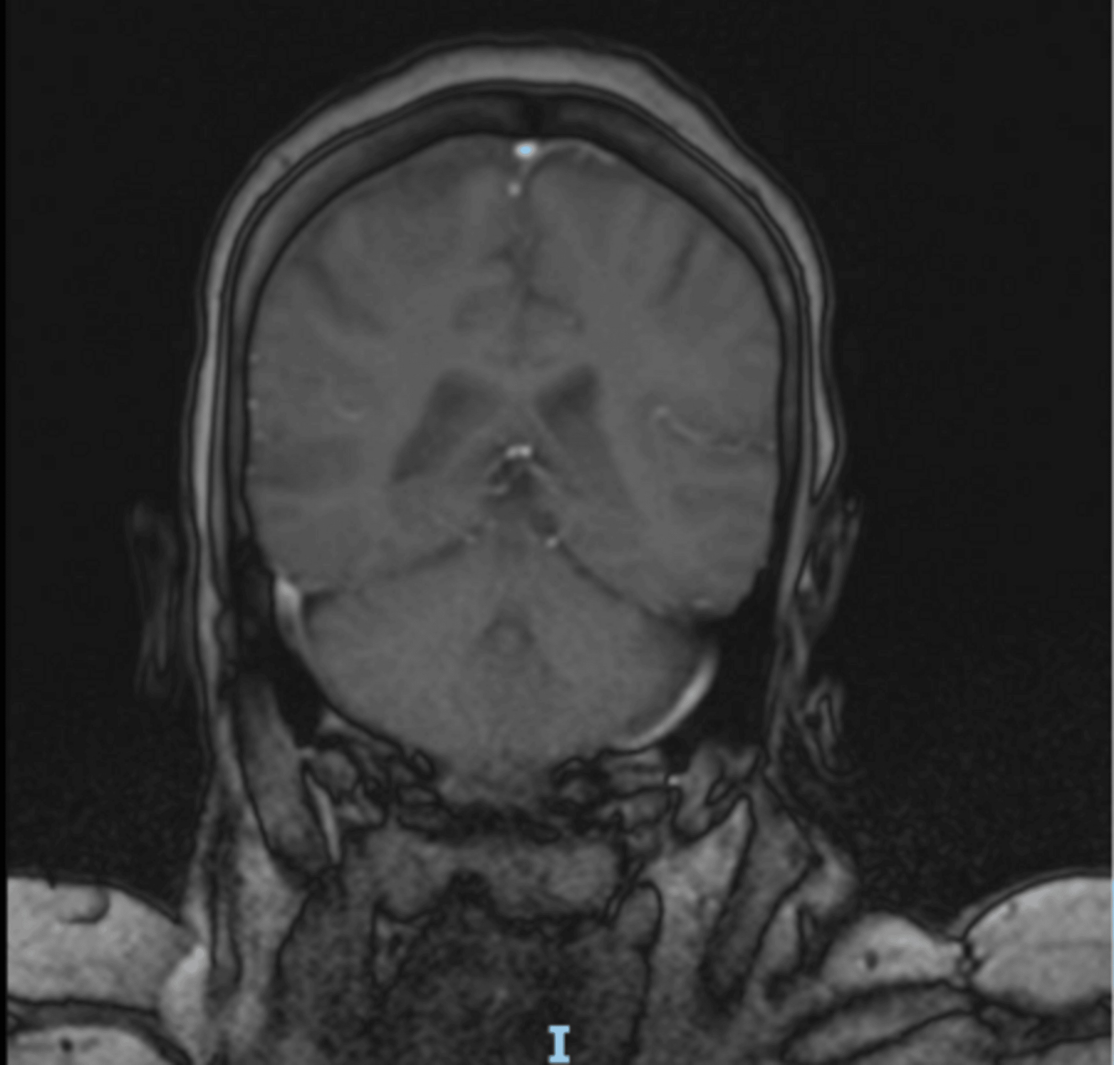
T1-weighted MRI brain with contrast in the coronal view with the basal ganglia in view
